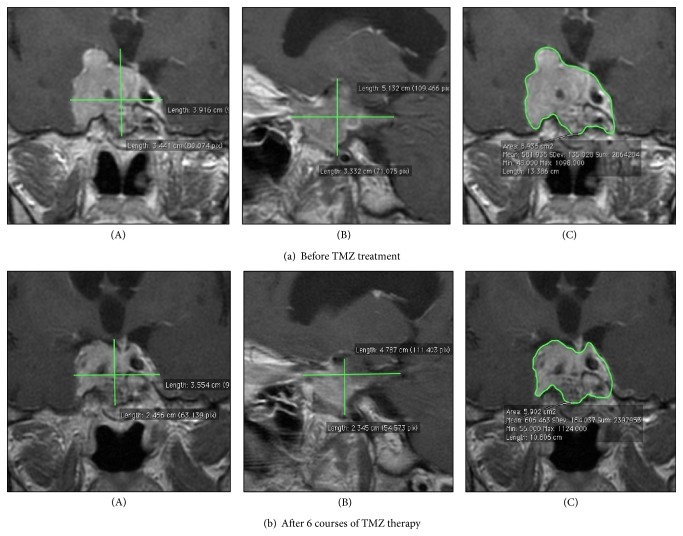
The assessment of tumour size (A, B) based on the MRI picture. The tumour volume (C) was measured using the computer program OsiriX. Frontal (A, C) and sagittal (B) scans.
